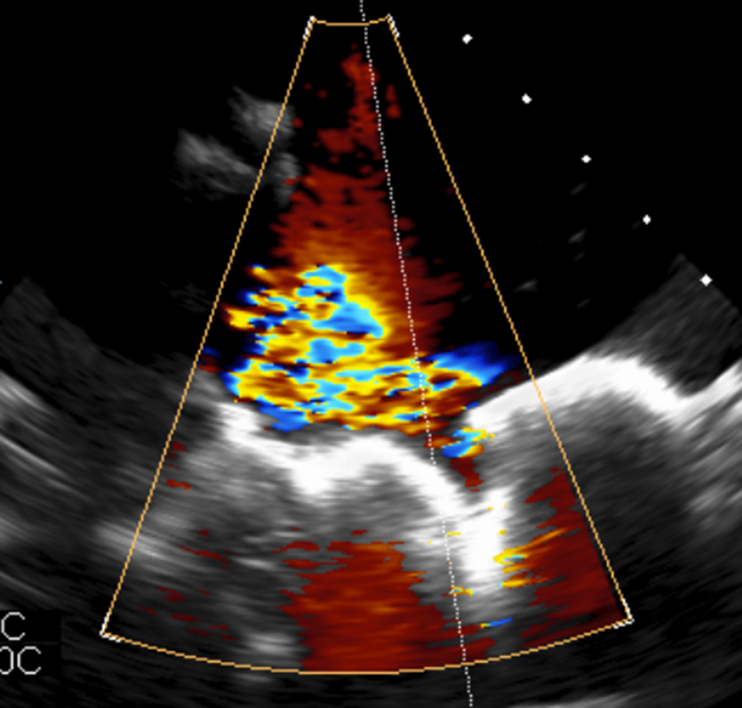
Validated P2 prolapse model showing characteristic regurgitant jet on ultrasound imaging.
